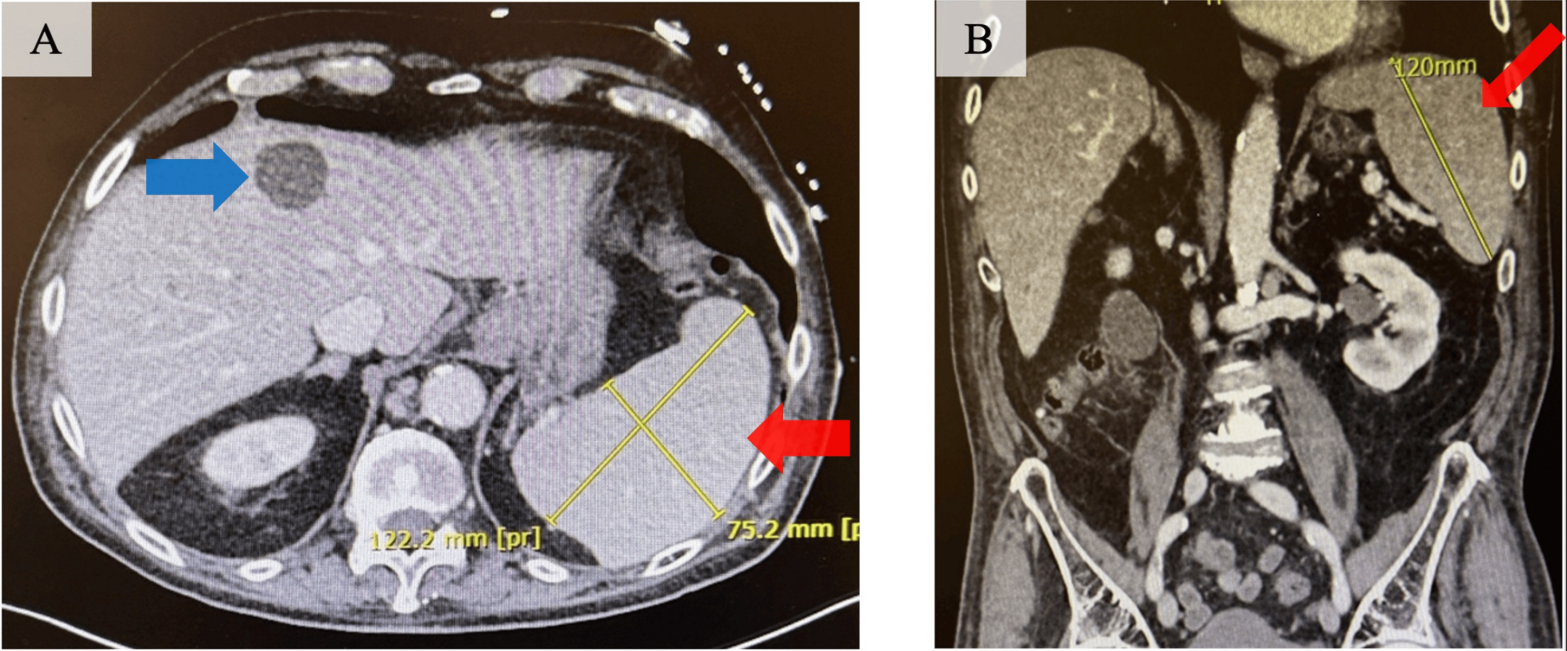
CT abdomen/pelvis with contrast (hospital day five) (A) Transverse image of the spleen and liver showing an enlarged spleen (red arrow) based on splenic index* of 1083, along with a stable hepatic hemangioma (blue arrow) present in previous imaging. (B) Coronal image of the abdomen showing splenic enlargement (red arrow). CT: Computed Tomography, *Splenic Index (SI) = spleen length × width × thickness Original clinical image; patient identifiers removed. Written informed consent for publication was obtained from the patient.
